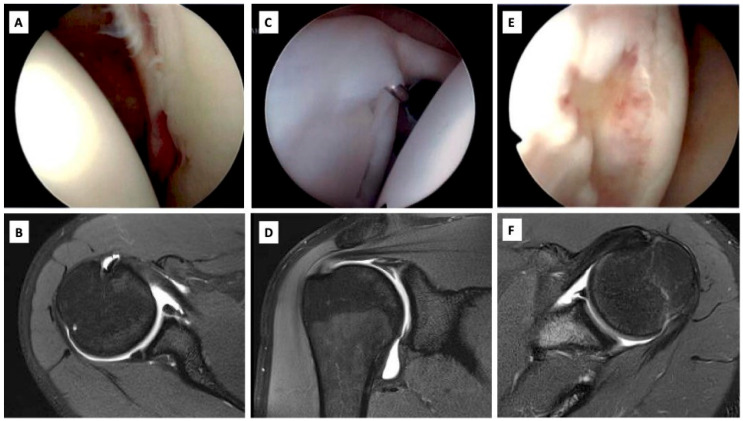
(A) Intraoperative image of Bankart lesion. (B) MR imaging of Bankart lesion. (C) Intraoperative image of SLAP tear. (D) MR imaging of SLAP tear. (E) Intraoperative image of Hill Sachs. (F) MR imaging of Hill–Sachs.
